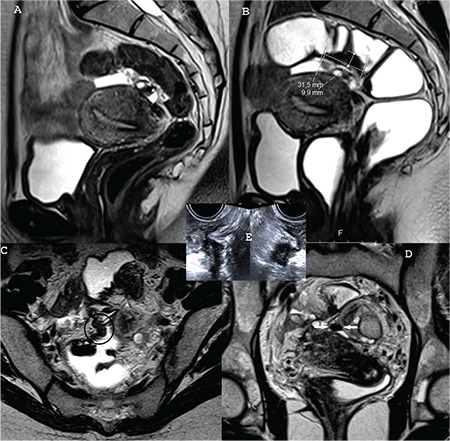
A) Sagittal T2W image of patient 4 in the present magnetic resonance imaging (MRI) scan obtained initially just before applying the rectal/vaginal opacification with water and the anti-peristaltic agent in our tertiary care hospital, but with the rectal cleansing enema, B) Sagittal T2W image of patient 4 in the present MRI obtained with the rectal/vaginal opacification with water and the anti-peristaltic agent use after the lesion is measured, C) Axial T2W image of patient 4 in the present MRI obtained with the rectal/ vaginal opacification with water and the anti-peristaltic agent use. The lesion is in the circle, D) Coronal T2W image of patient 4 in the present MRI obtained with the rectal/vaginal opacification with water and the anti-peristaltic agent use. The lesion is between the arrows, E) Transvaginal ultrasound in patient 4. The endometriotic intestinal nodule is measured. There is irregular thickening in the muscular propria
